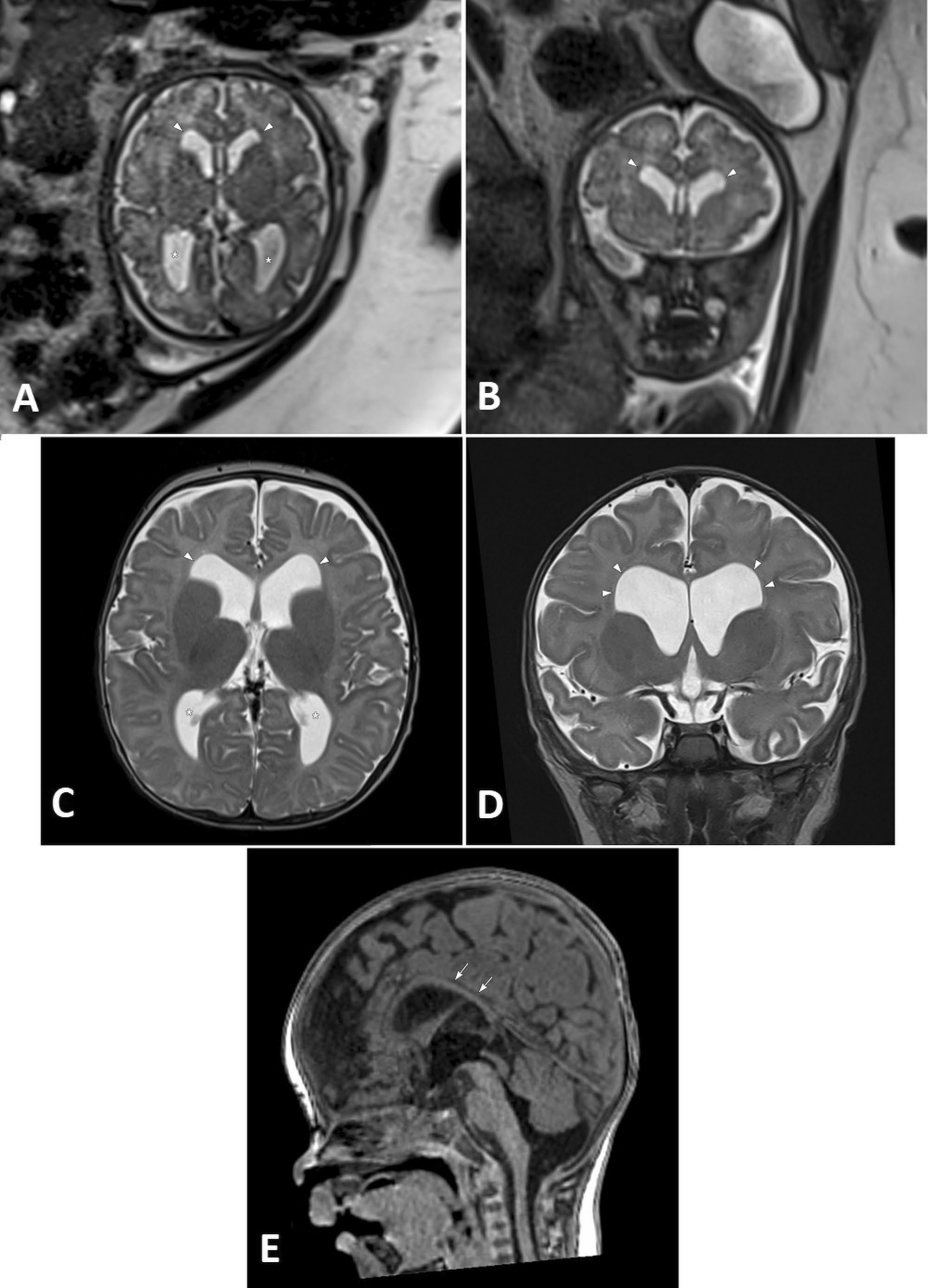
Axial (A) and coronal (B) T2‐weighted HASTE fetal brain MRI at 35 weeks GA: Bilateral and symmetric mild dilatation of the lateral ventricles (white asterisks) and square‐shaped frontal horns (white arrowheads). Axial (C) and coronal (D) T2‐weighted spin‐echo brain MRI at 2 months: Mild bilateral dilatation of the lateral ventricles (white asterisks) with preferential anterior enlargement and square‐shaped frontal horns (white arrowheads). Sagittal reformat 3D T1‐weighted MPRAGE sequence (E): Thin corpus callosum (white arrows).
